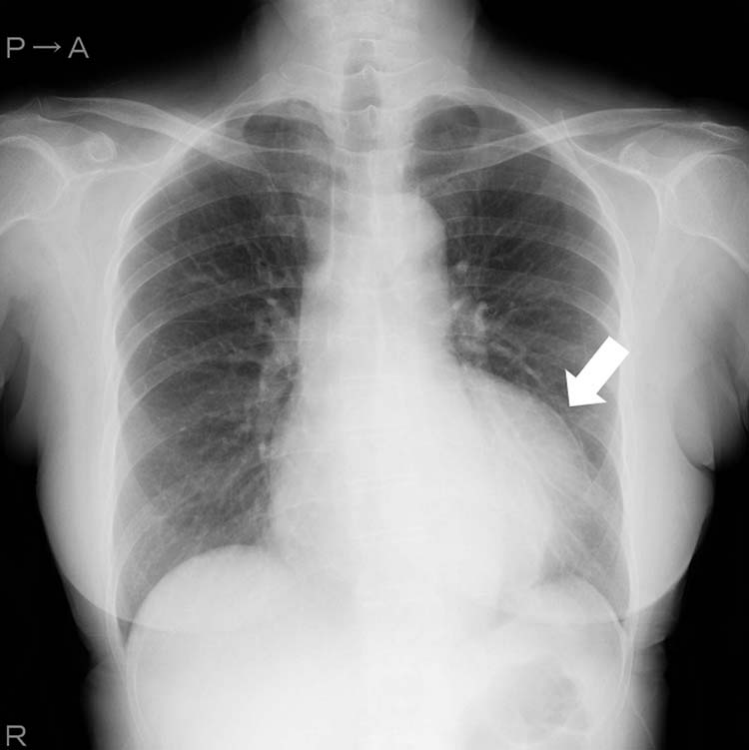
Chest radiography shows aneurysm as protruding mass.
